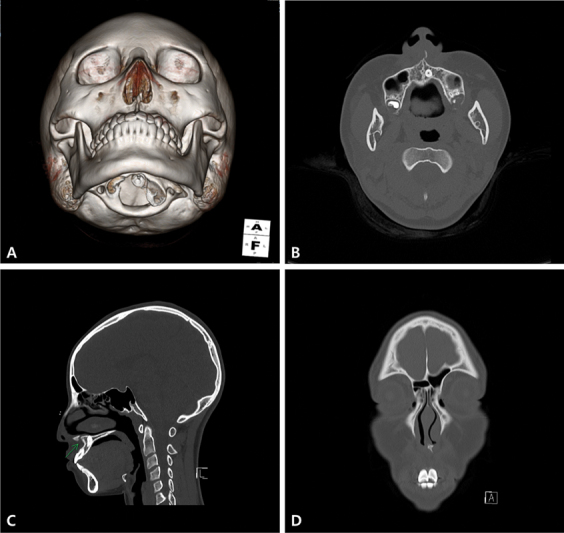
Preoperative computed tomography (CT) scan with three-dimensional (3D) reconstructions. Note that the anterior nasal spine was fractured and displaced to the left. (
